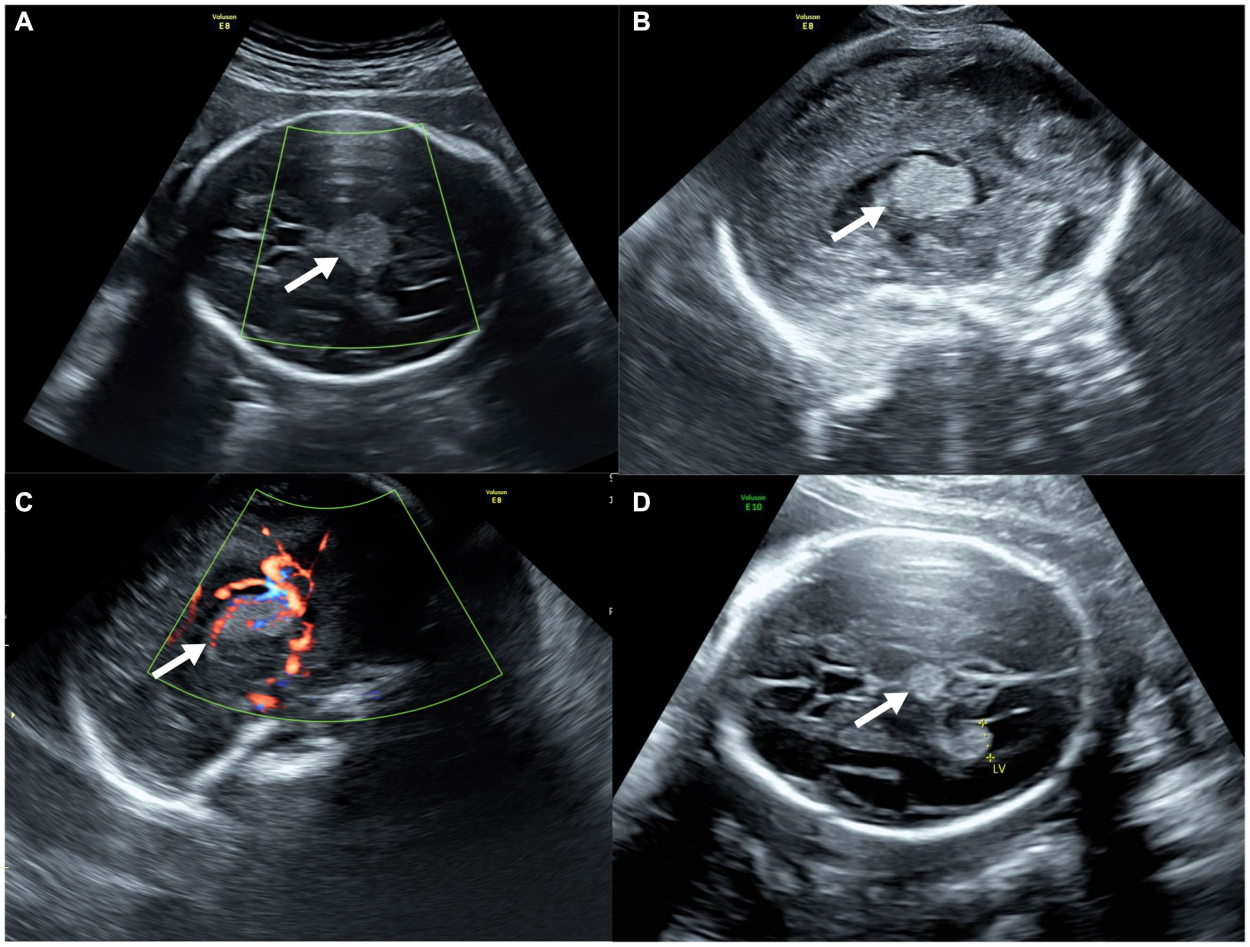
Prenatal ultrasound of fetal intracranial medulloepithelioma. (A) CDFI through the transabdominal approach; (B,C) sagittal view of the tumor through the transvaginal approach without (B) and with (C) CDFI; (D) ultrasound of the tumor at 23 weeks of gestation (axial view).
